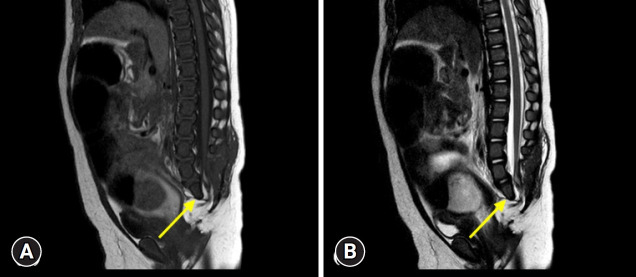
Sagittal magnetic resonance imaging of the lumbosacral spine. T1-weighted (A) and T2-weighted images (B) show a hypoplastic S2 vertebra (yellow arrows) as well as agenesis of the lower sacrum and coccyx.
